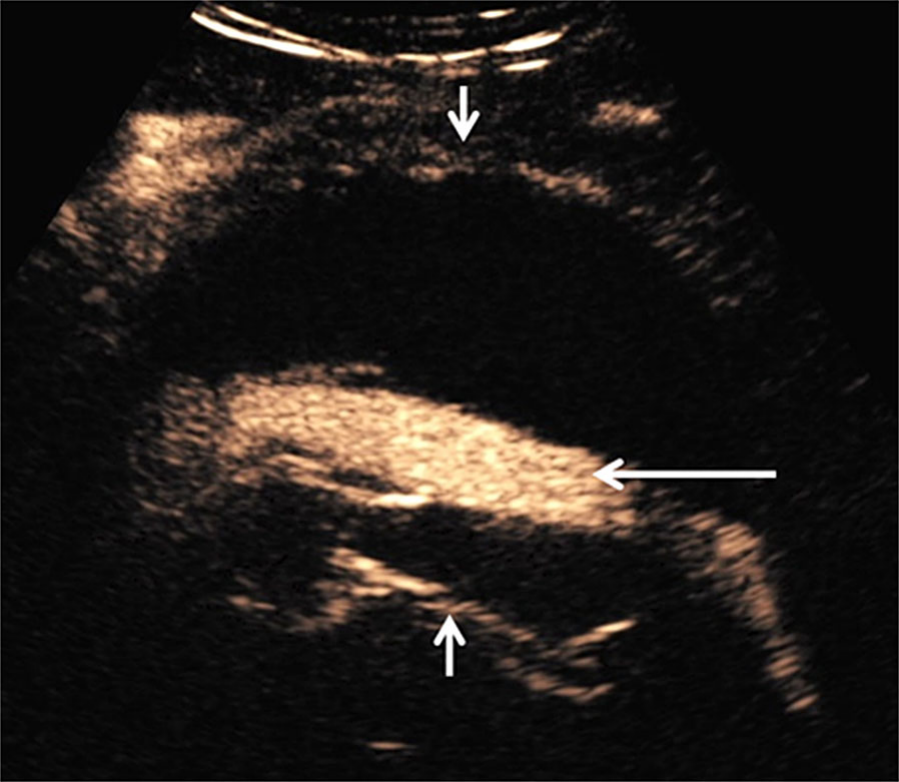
Routine post-elective EVAR follow-up scan from a 83-year-old man showed an expanding aneurysmal sac. CEUS was performed to look for endoleak. Longitudinal view of an aortic stent graft within the dilated aneurysm sac (between short arrows). CEUS image demonstrates microbubble ultrasound contrast within the patent aortic stent graft with no evidence of “endoleak” (long arrow)
